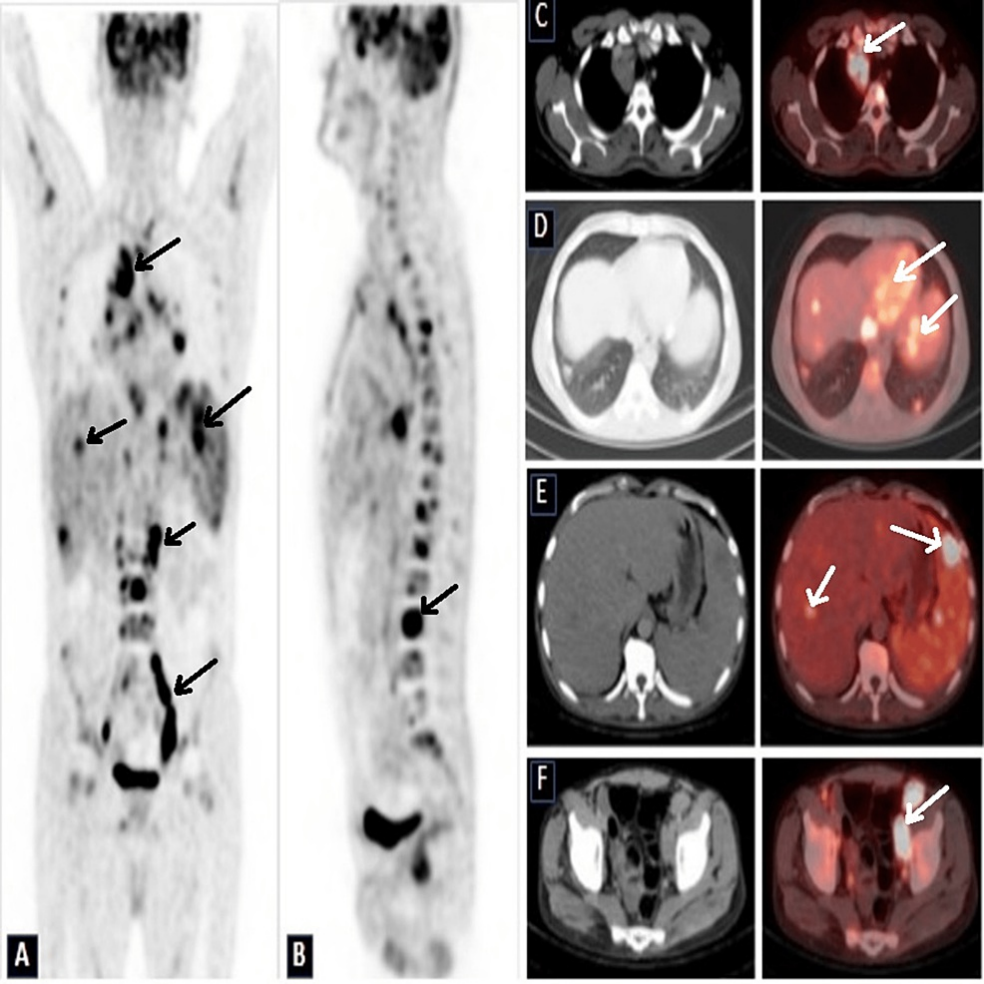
Coronal (A) and sagittal (B) maximum intensity projection (MIP) views of baseline F18-FDG PET-CT showing hypermetabolic nodal disease above and below the diaphragm with hepatosplenic and marrow involvement. C: Axial CT and fusion PET-CT images at different levels show F18-FDG avid mediastinal nodes, D: Bilateral pulmonary nodules, E: Hepatosplenic hypodensities, F: Pelvic lymphadenopathy PET-CT: Positron emission tomography-computed tomography, F18-FDG: Fludeoxyglucose F18
